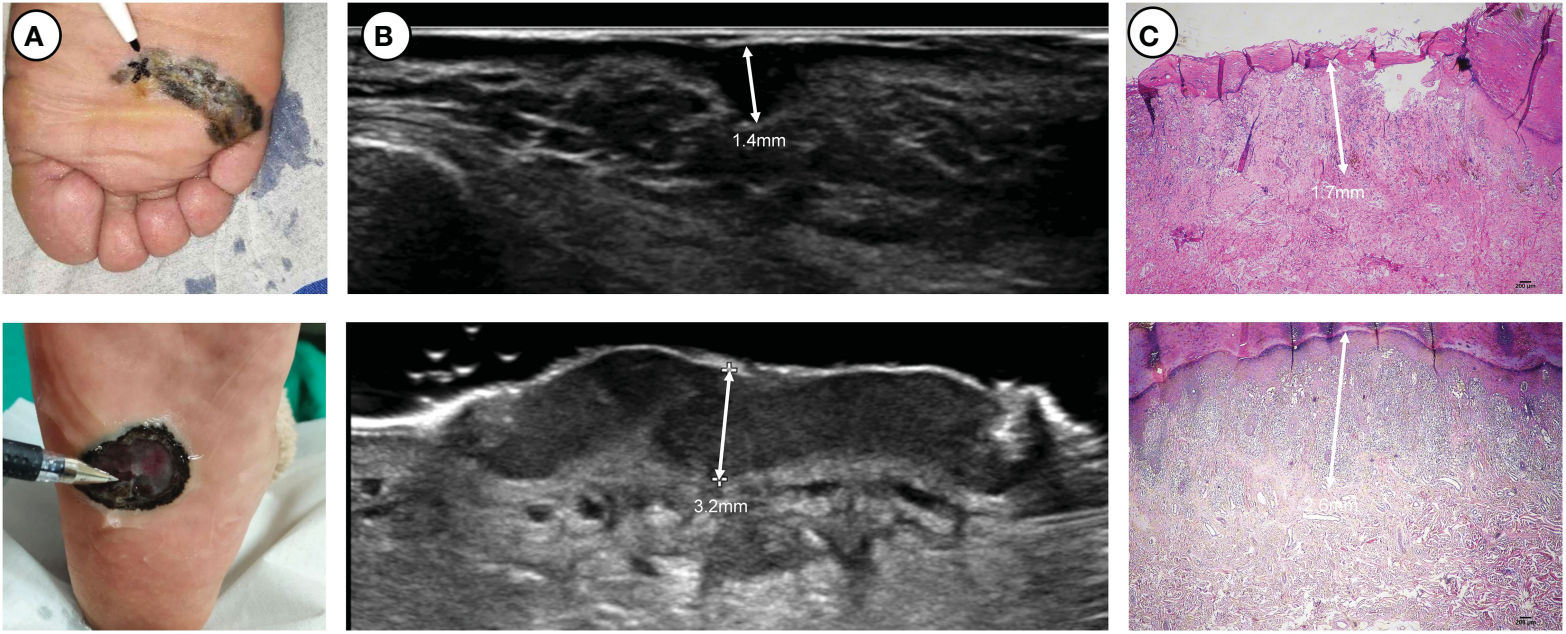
Two thick ulcerated nodular melanomas located on the plantar side: (A) clinical pictures, (B) ultrasound pictures and (C) pathological pictures. At the apex of the pen lies the location where ultrasonic measurements exhibit the maximum thickness.
